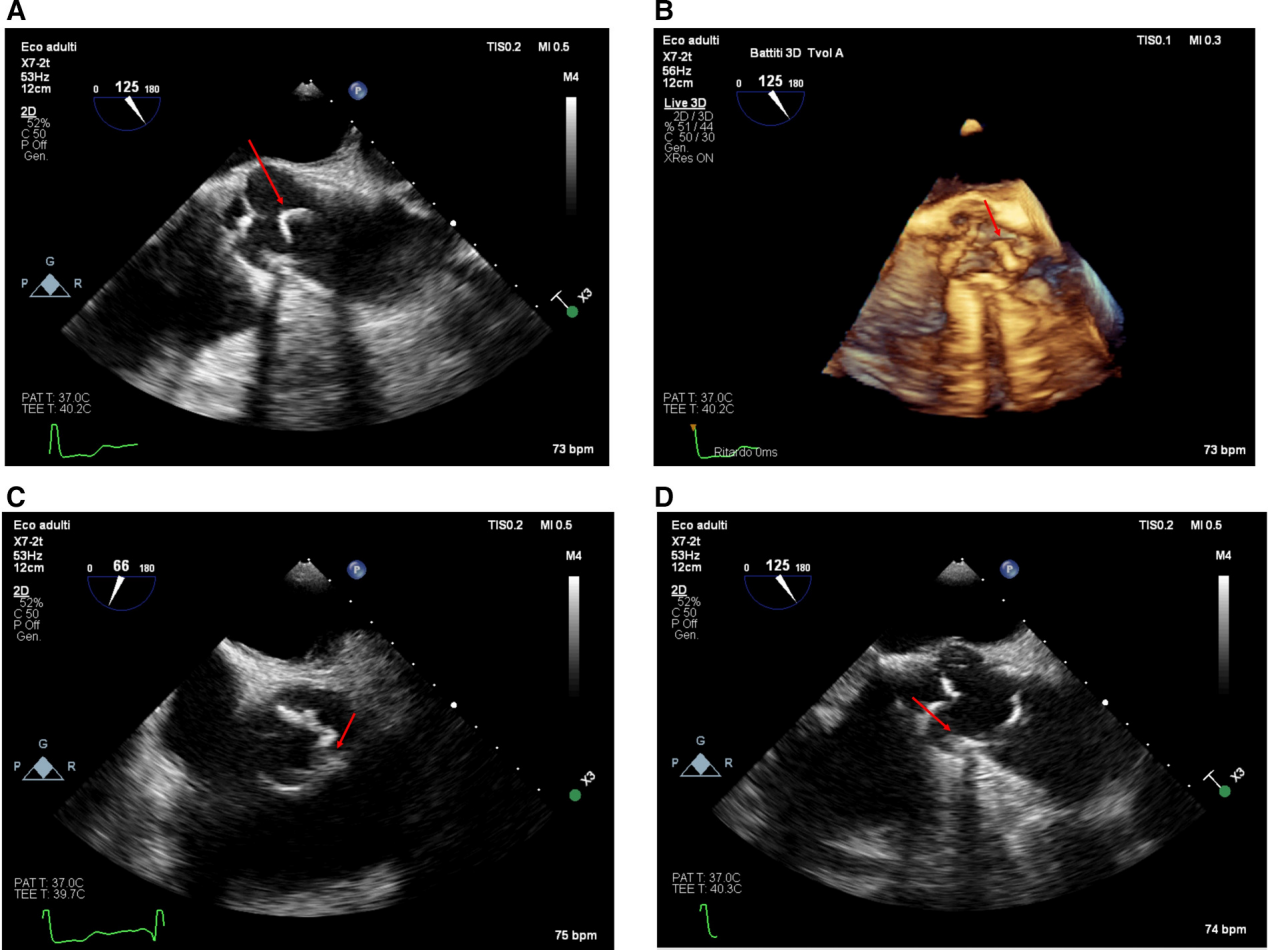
The appearance of the mass from the transesophageal approach at the mid-esophageal 120-degree view (ME AV LAX view) in 2D [(A), upper left panel, taken from Supplementary Video S2] and with a real-time 3D reconstruction [(B), upper right panel, Supplementary Video S3–S4]. The lesion appeared from the transesophageal approach in the mid-esophageal short-axis view at 66° [(C), lower left panel] and the 125° long-axis view [(D), lower right panel]. The calcified base of the plaque is visible (red arrow); notice the shadow cone [(D), lower right panel—red star] arising from the calcified plaque in the long-axis view.
